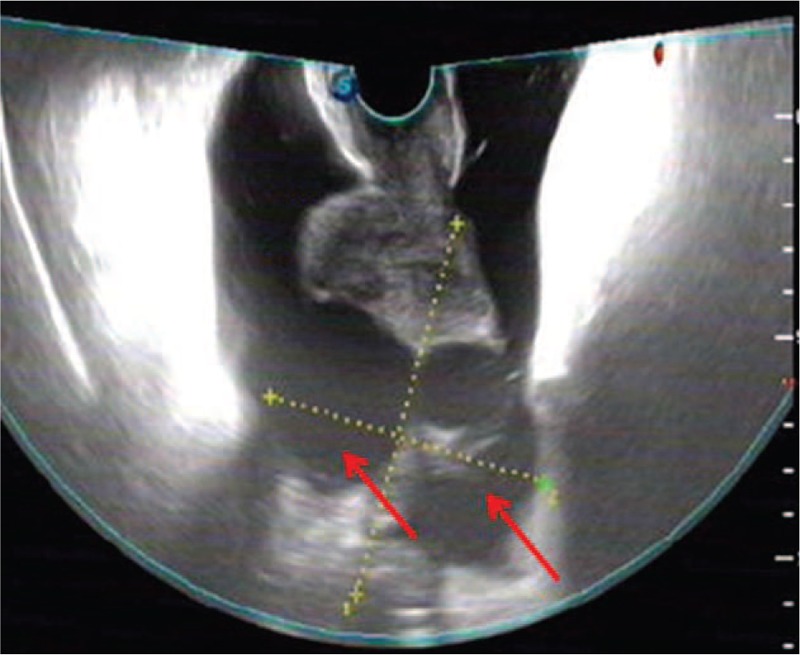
Gynecologic sonography showing an 8.8 × 7.5 cm mass (red arrow) with mixed density and irregular shape behind the uterus.
